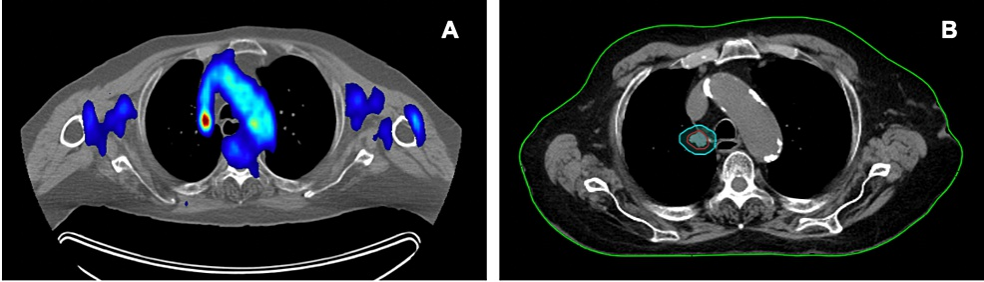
Tumor location and target contours Location of ultra-central lung nodule on PET/CT (A) at time of decision to undergo treatment. Target contours with gross tumor volume (GTV) in red and planning target volume (PTV) in cyan (B).
